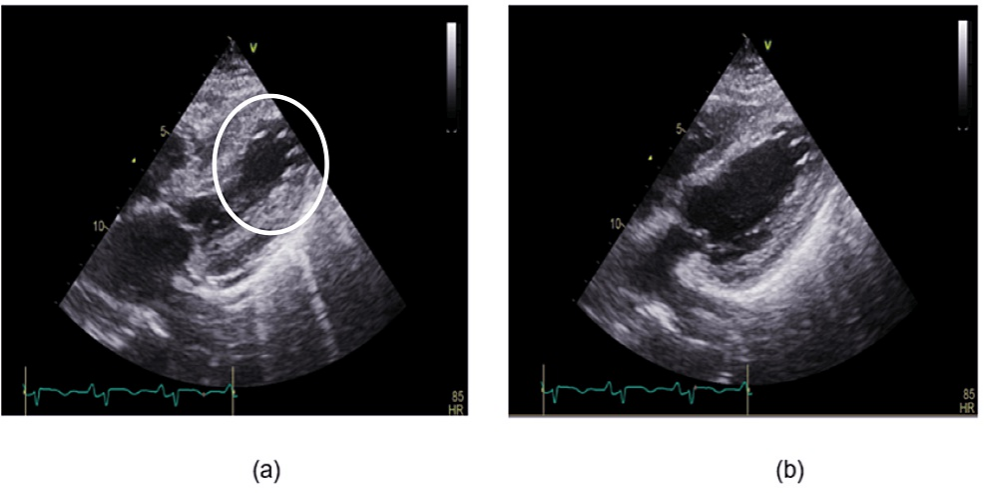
Echocardiogram showing ballooning of the apex observed during systole (a) (White circle) compared to diastole (b)
